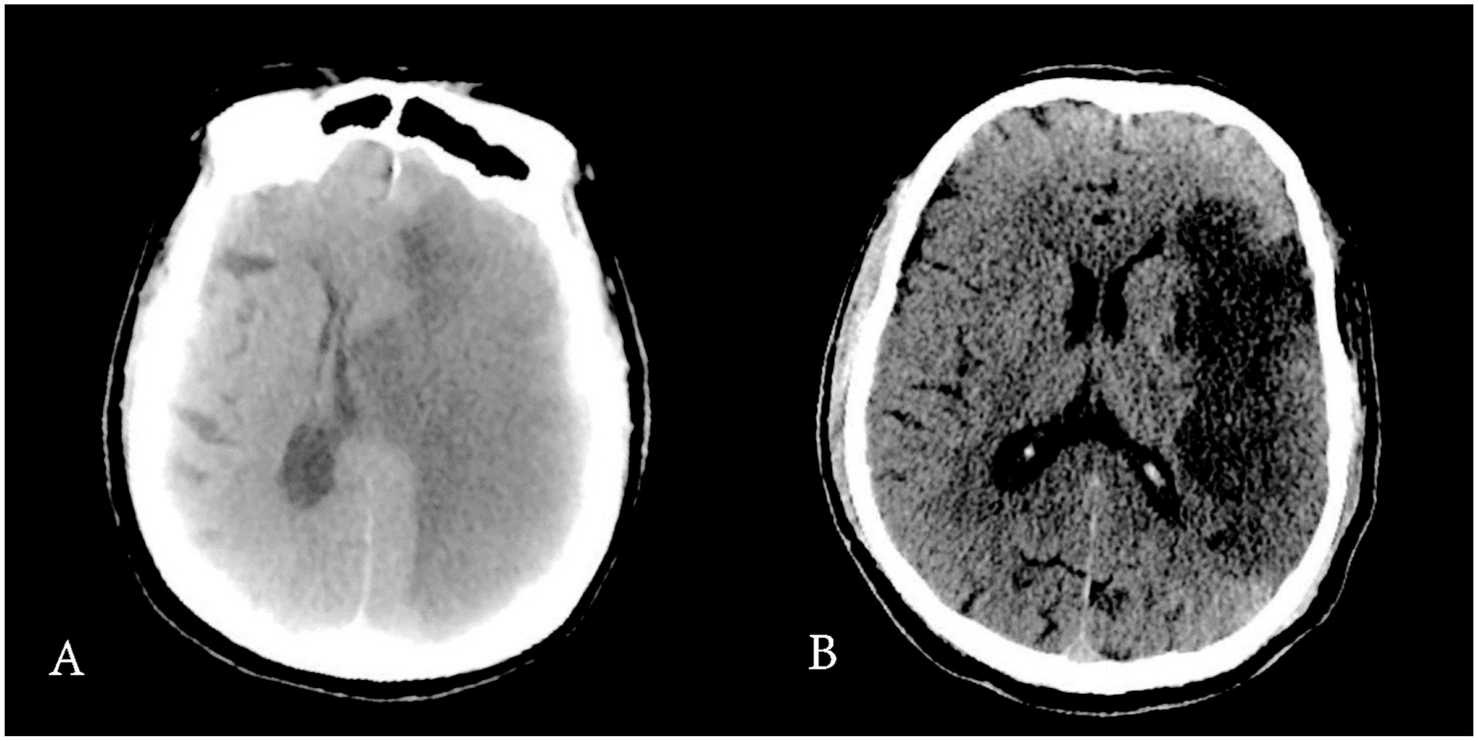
CT scan of MCE and non-MCE. (A) Refers to a MCE patient, (B) refers to a non-MCE patient.
